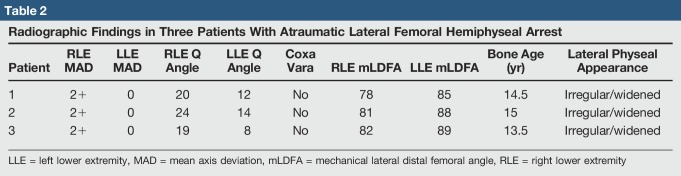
Radiographic Findings in Three Patients With Atraumatic Lateral Femoral Hemiphyseal Arrest
